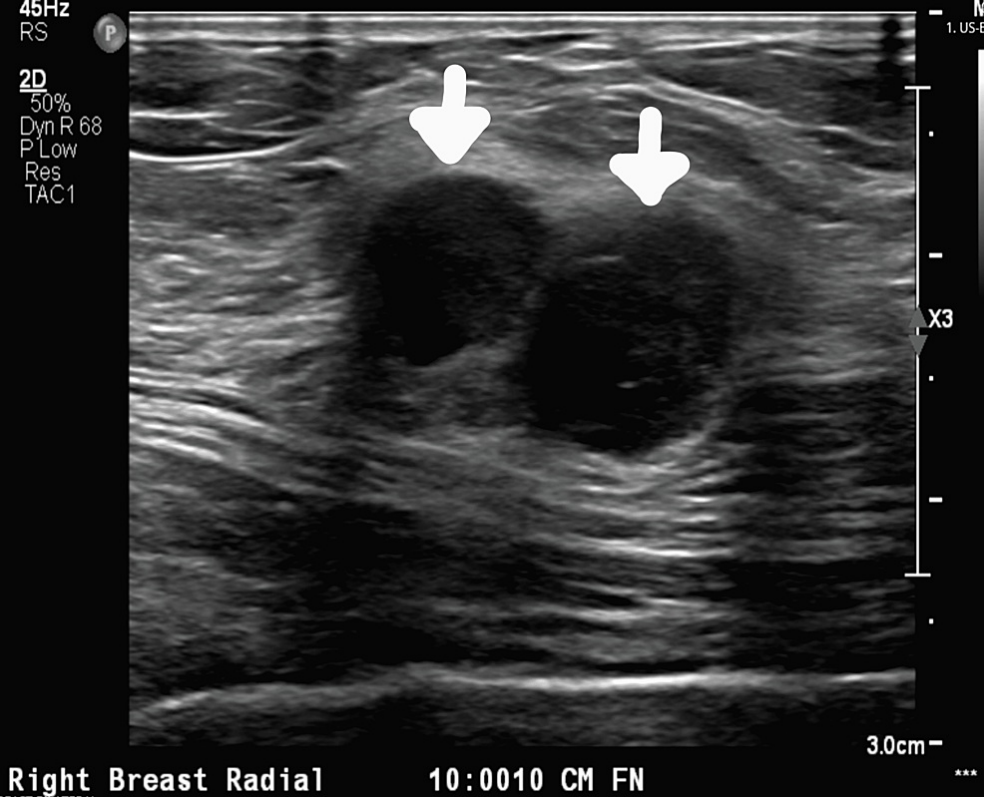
Follow-up ultrasound A bilobed 2x1.6 cm mass at the 10 o’clock position 10 cm from the nipple
